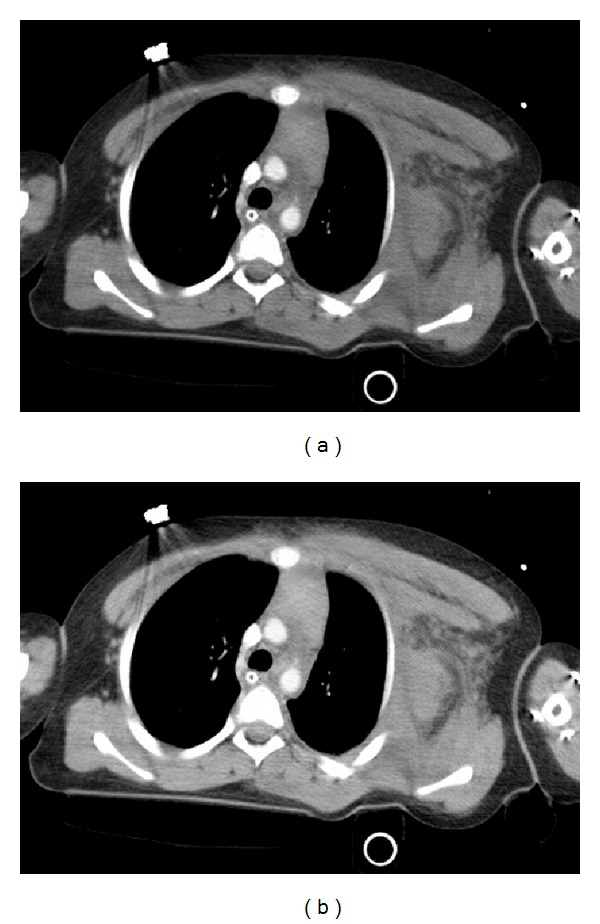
CT scan of the chest shows left apical pulmonary contusion with soft tissue edema, subcutaneous fat infiltrations, and muscular thickening with gas in the subclavicular space.
